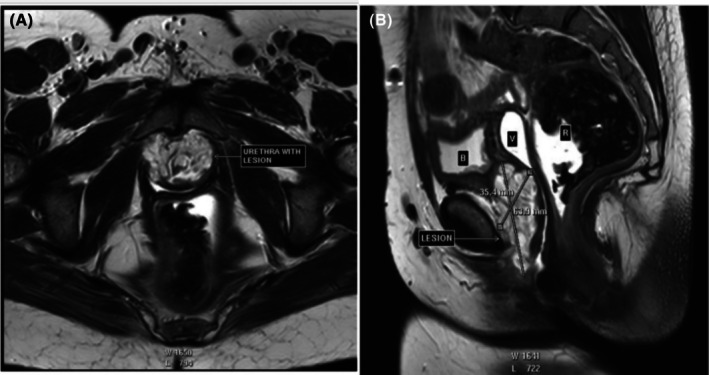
(A) Showing MRI images of the urethral lesion and (B) the surrounding pelvic organs.
